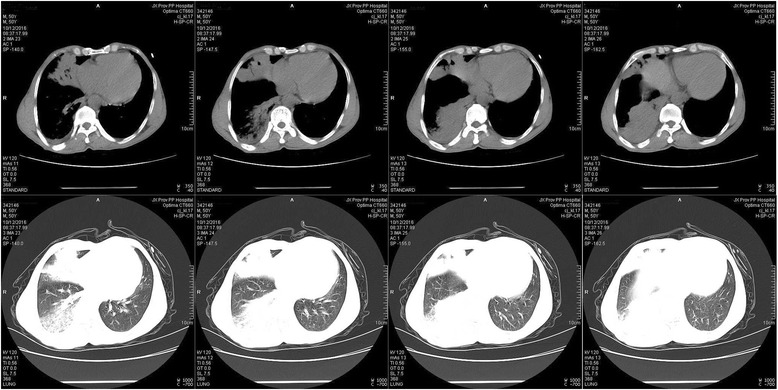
The first Chest CT (Oct. 12) showed a spot-like, high-density shadow enlarged as before
